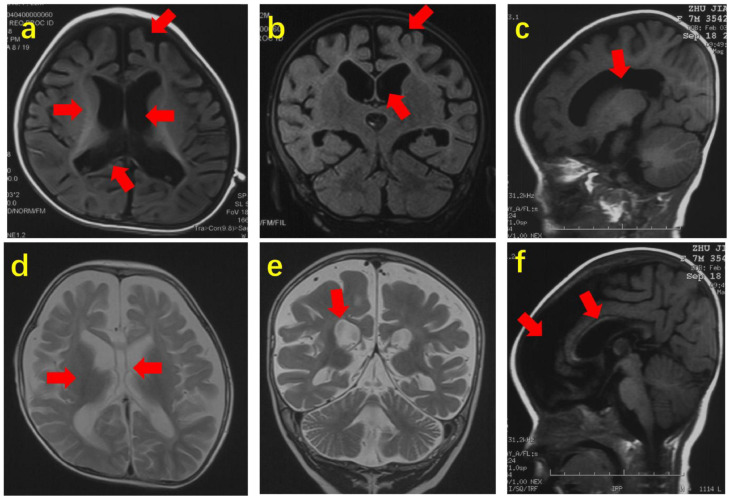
Brain MRI abnormalities in patients with SCN2A variants. (a,b) Axial and coronal T1-weighted images (Patient 14, age 22 months) showing corpus callosum agenesis, delayed myelination, frontotemporal dysplasia, and ventricular enlargement. (c,f) Sagittal T1-weighted images (Patient 29, age 7 months) showing similar findings. (d,e) Axial and coronal T2-weighted images (Patient 39, age 12 months) showing corpus callosum agenesis, delayed myelination, and ventricular enlargement. Arrows indicate lesion sites. Taken from Zeng et al. [51] under the terms of the Creative Commons Attribution License (CC BY).
